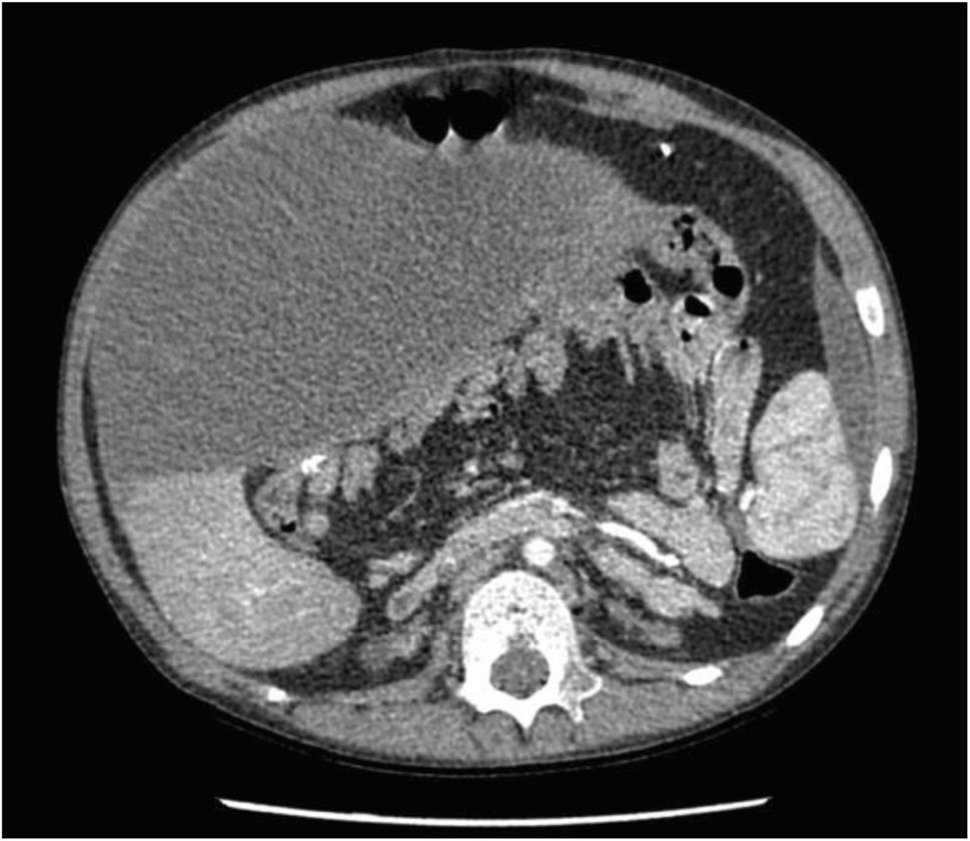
Pre-operative CT scan of *Case 4* showing a large encapsulated abdominal fluid collection with associated peripheral peritoneal calcification
